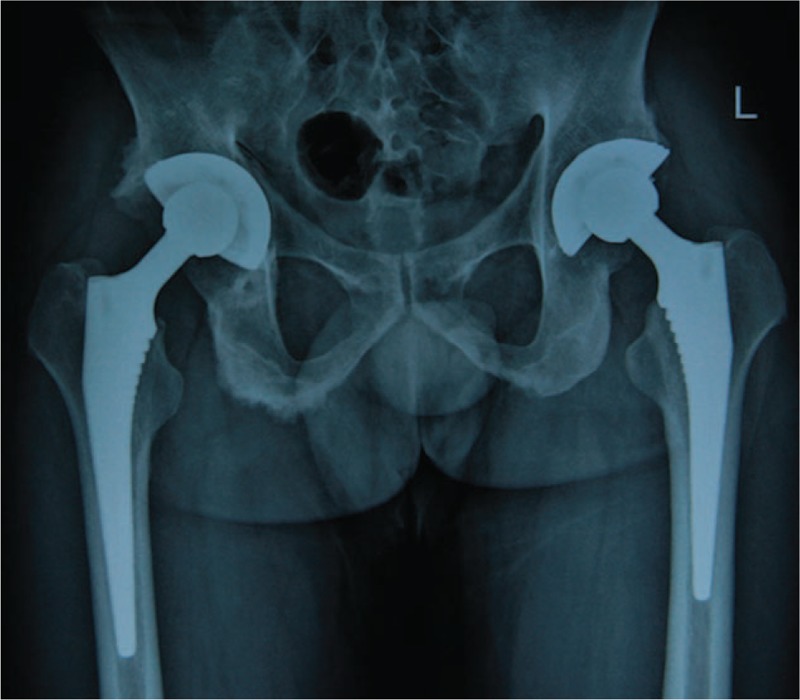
Postoperative anteroposterior radiographs of the patient 4 years after cementless THA. THA = total hip arthroplasty.
